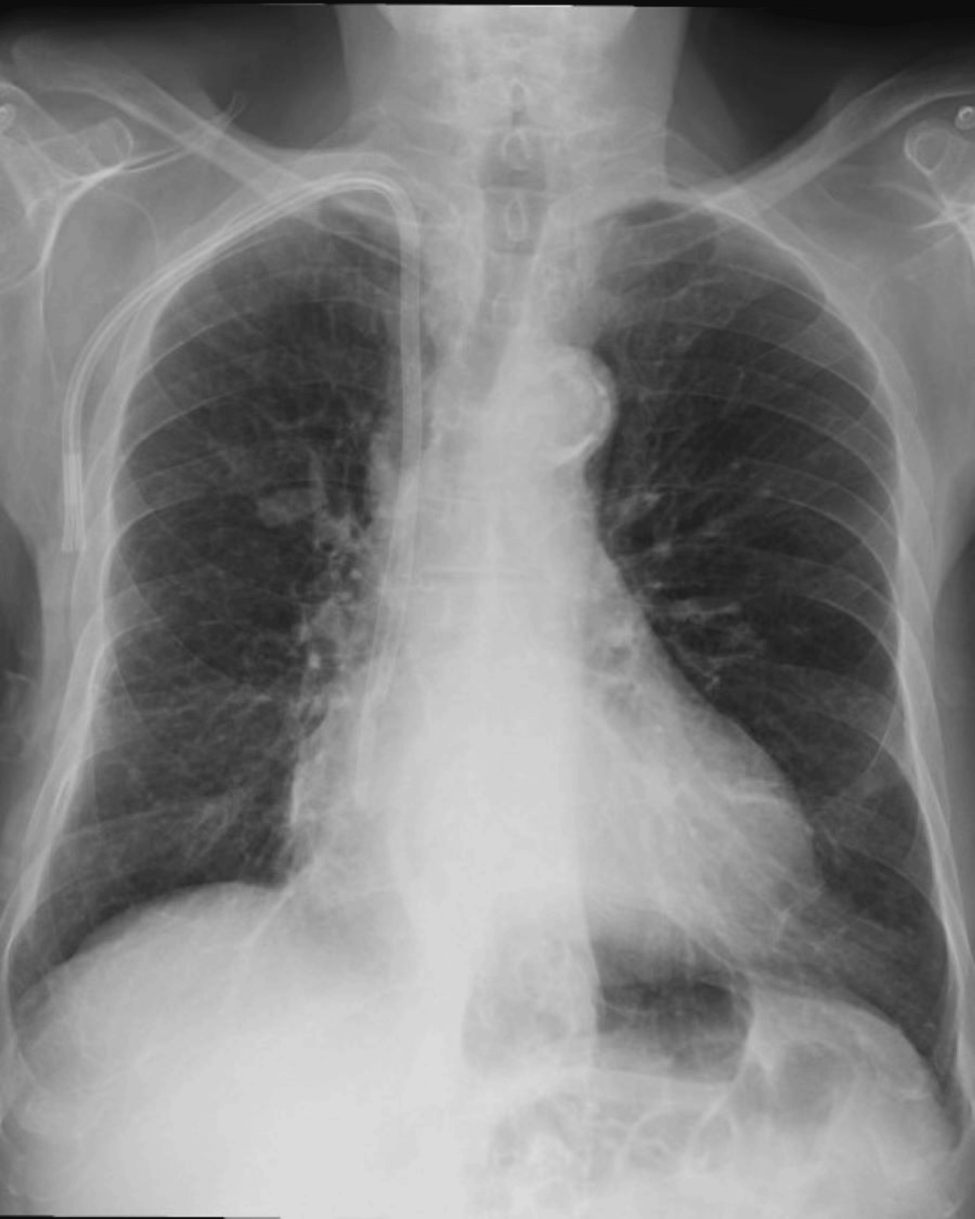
Chest X-ray after diuretic therapy
